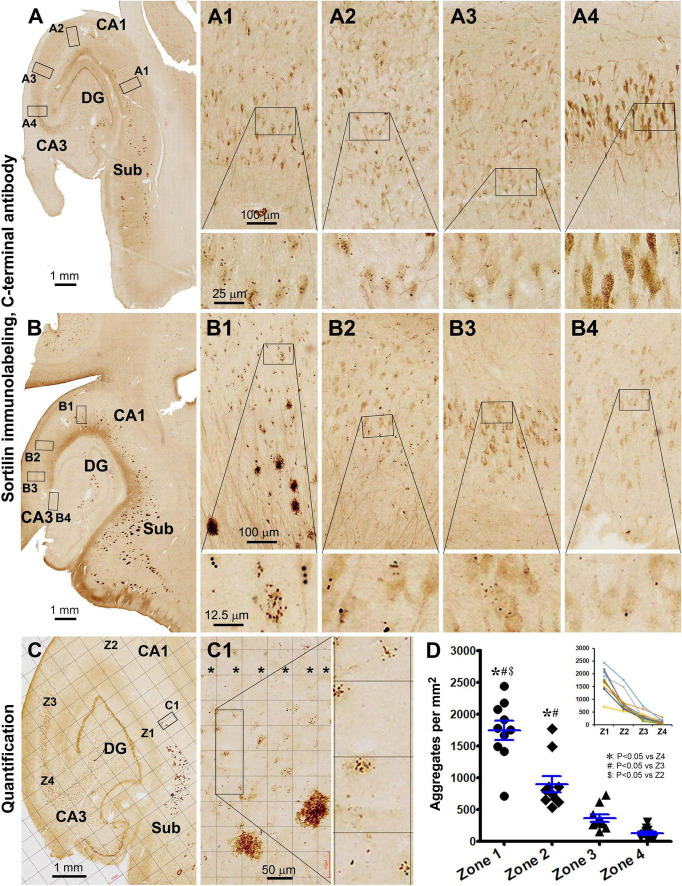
Quantitative analysis of the regional density of intraneuronal sortilin aggregates using temporal lobe sections immunolabeled with the CT sortilin antibody. [(A–A4) Are from case #49; (B–B4) from case #39, and (C–C1) from case #51]. A trend of regional expansion of sorfra plaques in the stratum pyramidale (s.p.) from the subicular to CA1, CA2, and CA3 subregions is noticeable by comparing the images from the three cases [(A–C)]. Note that the densities of intraneuronal aggregates are also reduced as moving from the subicular to CA3 direction [(A1–A4,B1–B4)]. (C) And enlarged views show a zone-based quantification detailed in “Materials and methods,” with areal densities of the aggregates in four zones (Z1–Z4, in C) across s.p. (* in width of 300 μm) are quantified on-screen at high resolution using the Motic viewer. (D) Summarizes the mean densities in the four zones obtained from 10 pAD/AD cases, with the inserted graph plotting the tendency lines in individual cases. Statistical testing results (one-way ANOVA) are marked, with *, # and $ symbols indicating the existence of difference. Abbreviations are as defined in Figure 1. Scale bars are as indicated.
